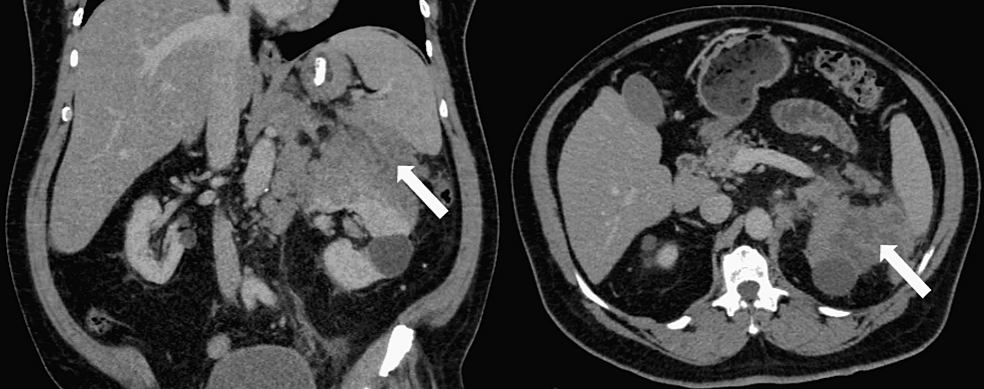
CT scan of abdomen and pelvis The images reveal a 10.8 x 8.9 x 8.2 cm irregular heterogeneous mass arising out of the superior pole of the left kidney with extension superiorly and invasion of the undersurface of the spleen. There is celiac axis adenopathy as well as retroperitoneal adenopathy and splenic hilum adenopathy CT: computed tomography
